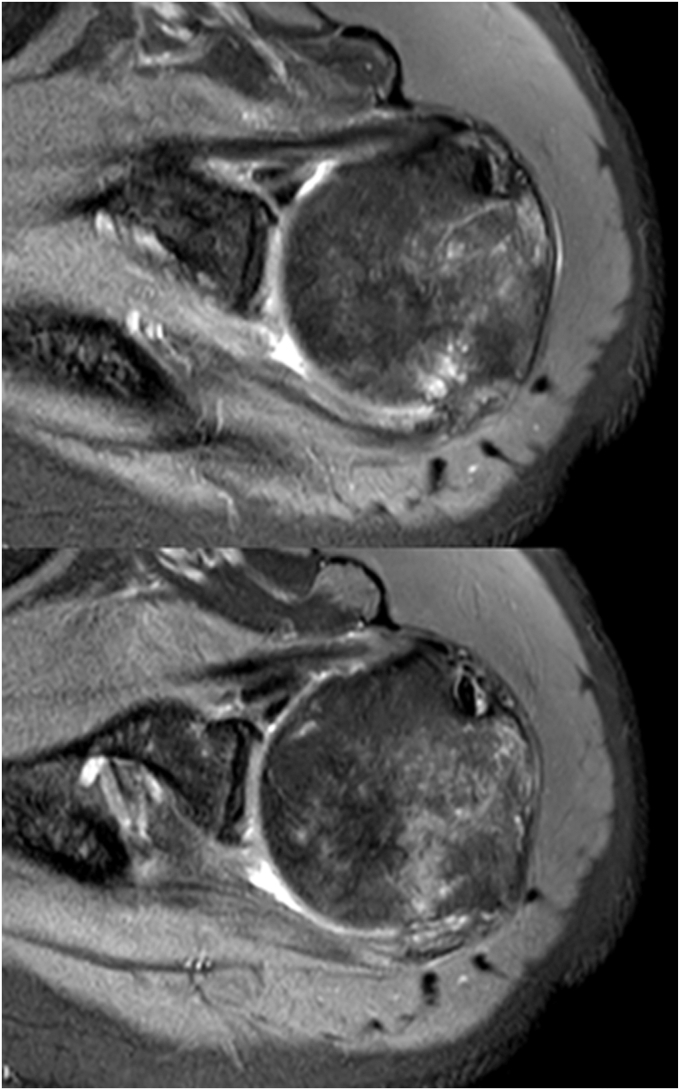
Magnetic resonance image performed preoperatively without intra-articular contrast (8 months and 9 days after the trauma).
